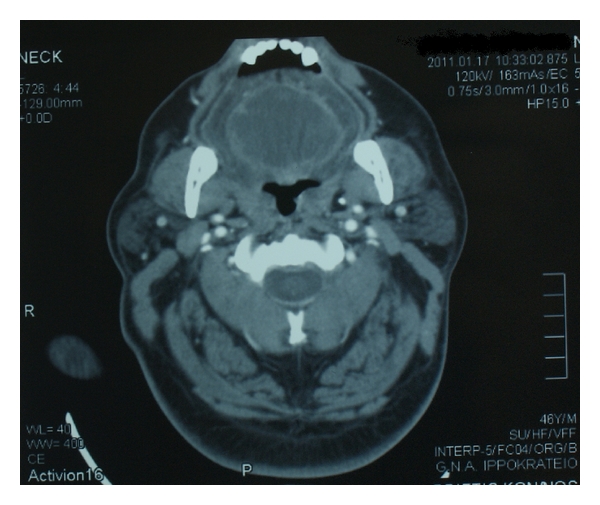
Computed tomographic evidence of a circumscribed mass within the tongue musculature, compatible with a lingual abscess.
